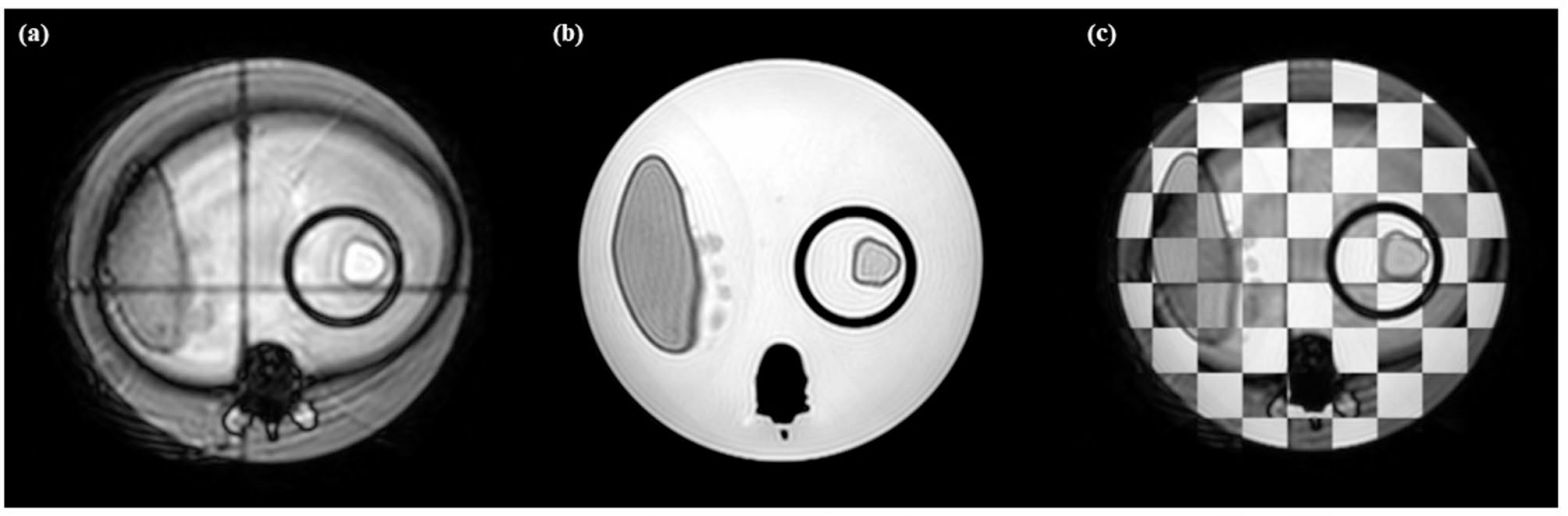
Manual image registration results of a (a) converted 2D cine MR image and (b) 3D T1 image, and (c) comparison between the two images as a checkerboard image using 3D Slicer.
